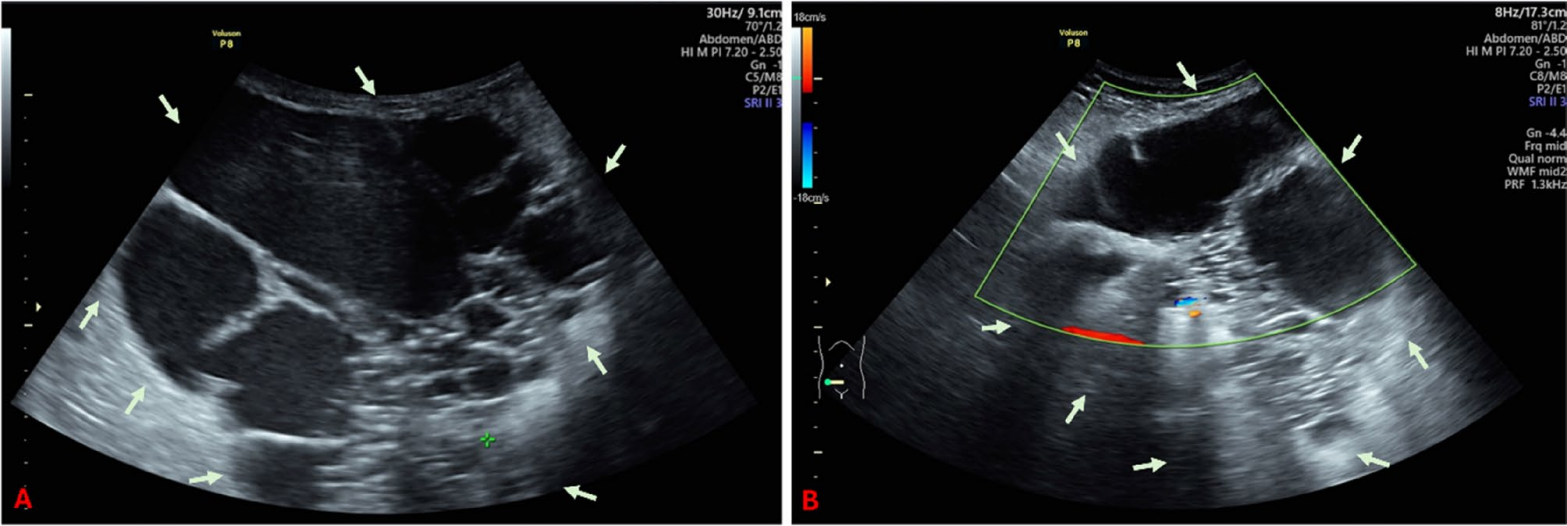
A: Transabdominal ultrasound image of the predominantly cystic abdominal wall tumor. B: Doppler sonographic image of the blood flow in the hyperechoic area of the tumor. The lesion is marked with arrows
